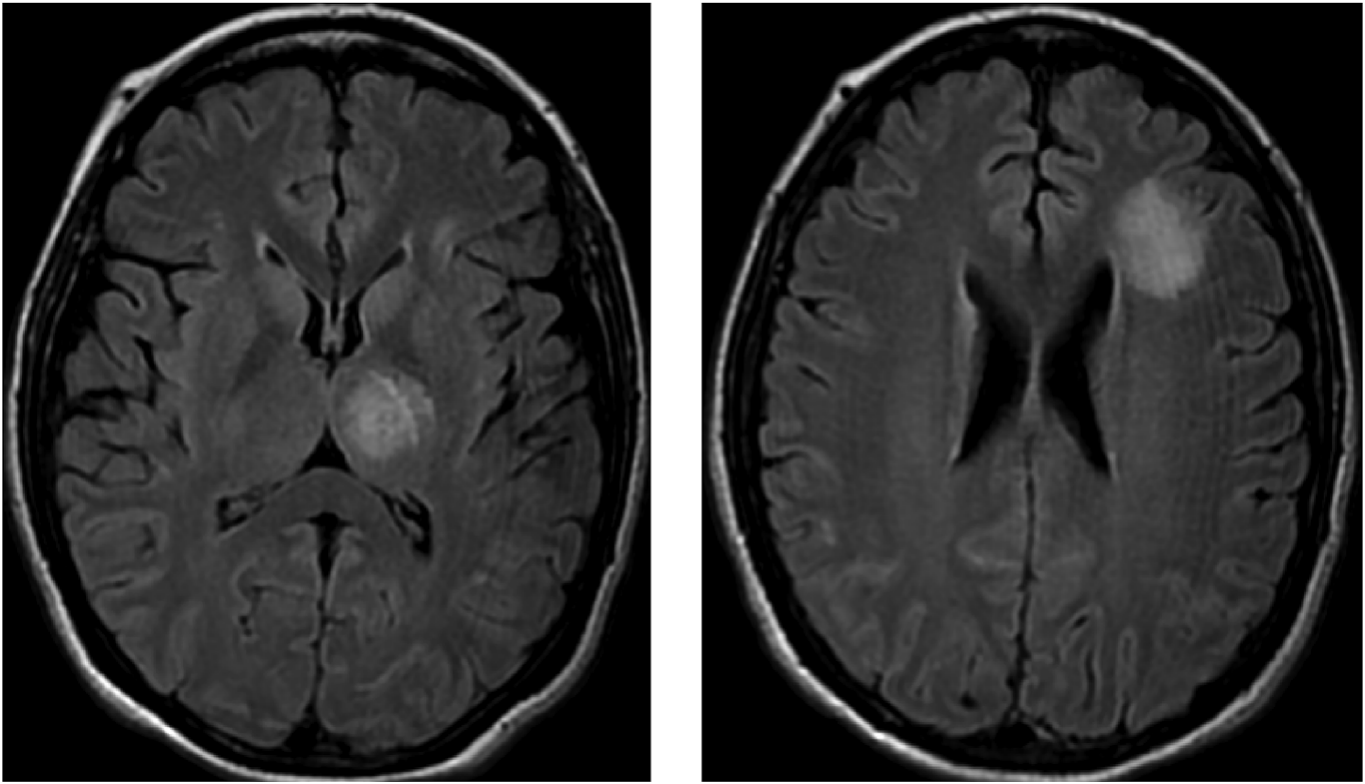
Brain MRI on HD 20. Left: thalamic lesion. Right: frontal lobe lesion.
